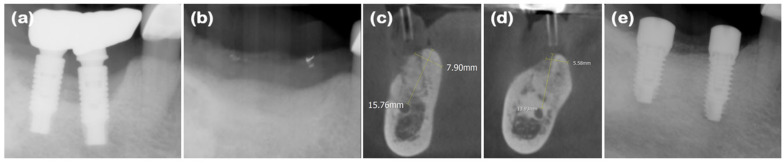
Radiographs of case 4. (a) The pre-operative periapical radiograph. (b) The post-operative periapical radiograph of the mandibular right molar areas, demonstrating the area filled with bone graft material. (c,d) CBCT images displaying the augmented site after healing. The alveolar ridge width was measured at approximately 7.90 and 5.58 mm on the mandibular first and second molar areas, respectively, which is sufficient for the placement of a standard diameter implant. (e) The periapical radiograph after the second implant surgery.
